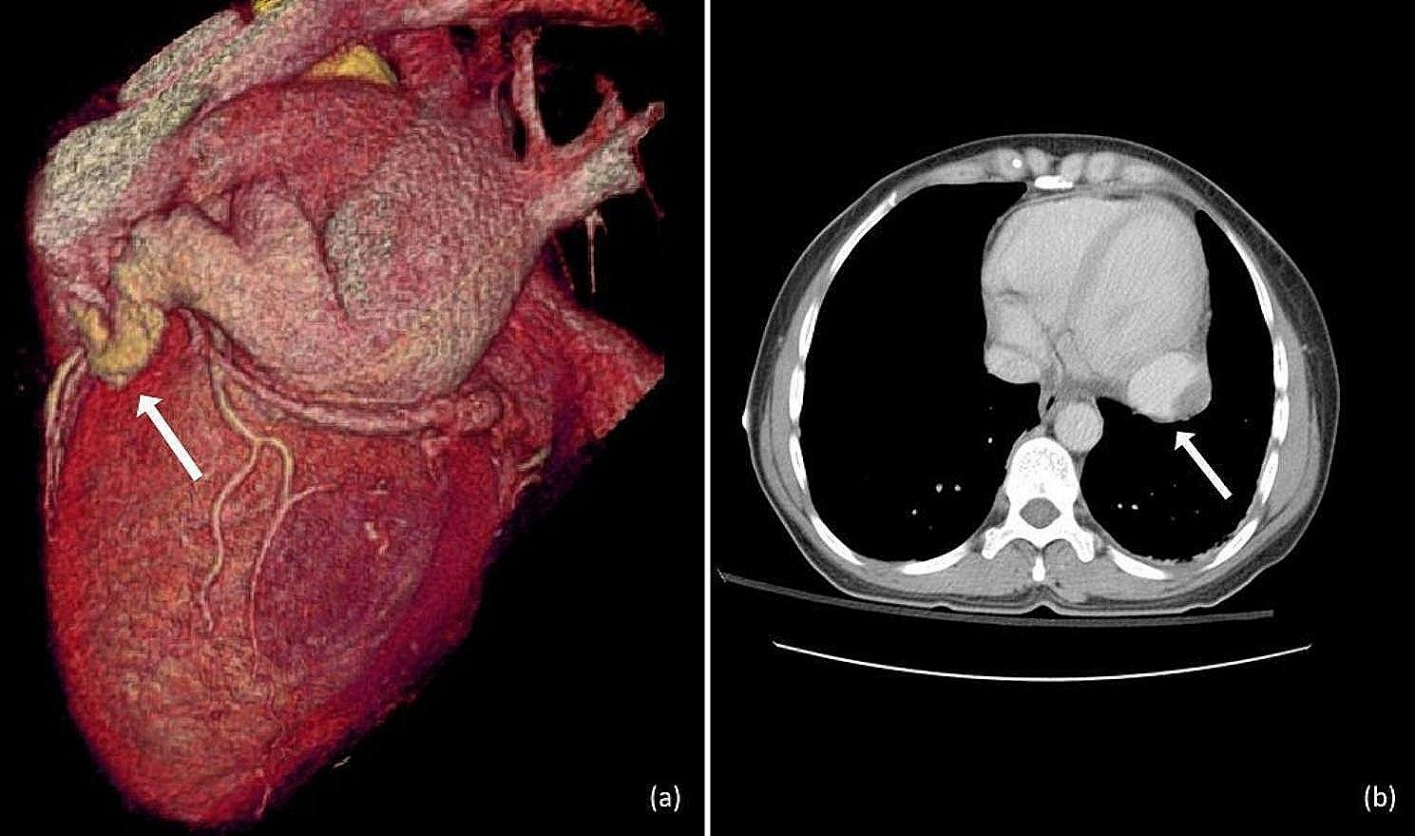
3D reconstruction (a) and depiction of the thrombus in the aneurysm through Computed Tomography (b)
